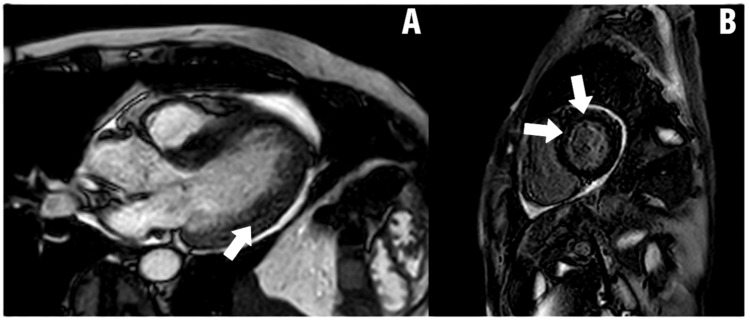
Cardiac MRI reveals acute peri-myocarditis. Arrows designate acute myocarditis involving the left ventricle. There is a diffuse mid myocardial delayed enhancement in all basal to apical segments along with pericardial enhancement. Moderate concentric left ventricular hypertrophy is also noted. According to the parametric mapping, there were significantly prolonged T2 and T1 mapping values. Myocardial native T1 time was 1250 ms and myocardial T2 time was 79 ms. (A) Horizontal long axis four chamber inferolateral wall view; (B) Short axis antero-apical and septal apical wall view Image Credit: Mark Zenker
